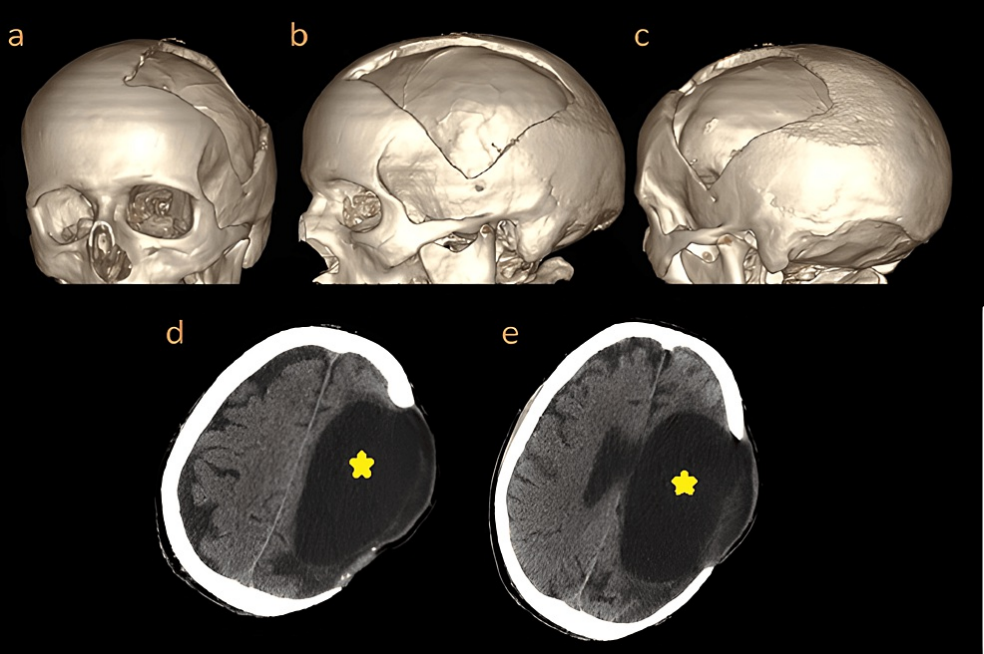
Two- and three-dimensional brain computed tomography a, b, c: The three-dimensional brain imaging was performed to allow the neurosurgical head-bone reconstruction. d, e: Two-dimensional brain computed tomography; the lateral ventricle on the left side (yellow star) appears to be more dilated in comparison with the previous brain imaging session.
